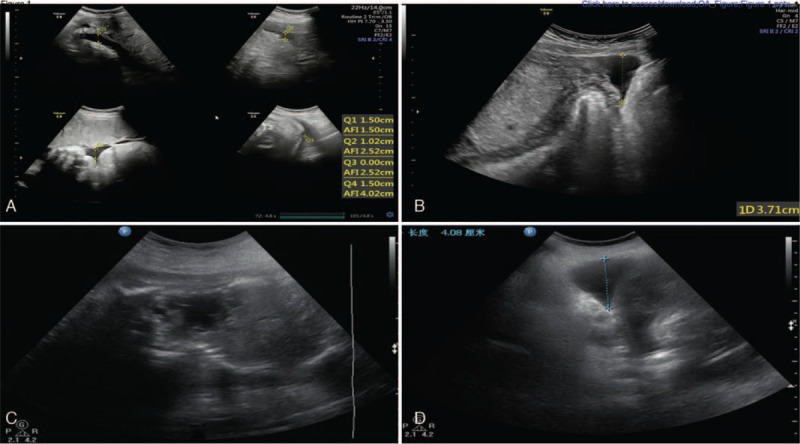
The sonographic findings of the patient with unscarred uterine rupture. The sonographic examination did not find any laceration in the uterine wall. Figure A and B were the results of transabdominal ultrasound performed on admission. Figure A demonstrated oligohydramnios; the amniotic fluid index was 4.02, and the maximum pool depth was 1.5 cm. Figure B revealed seroperitoneum; the maximum depth of abdominal effusion was 3.71 cm. Figure C and D were the results of repeated sonographic examination performed after admission. Figure C demonstrated there was almost no amniotic fluid in the amniotic cavity. Figure D revealed increased peritoneal fluid; the maximum depth of abdominal effusion is 4.08 cm.
